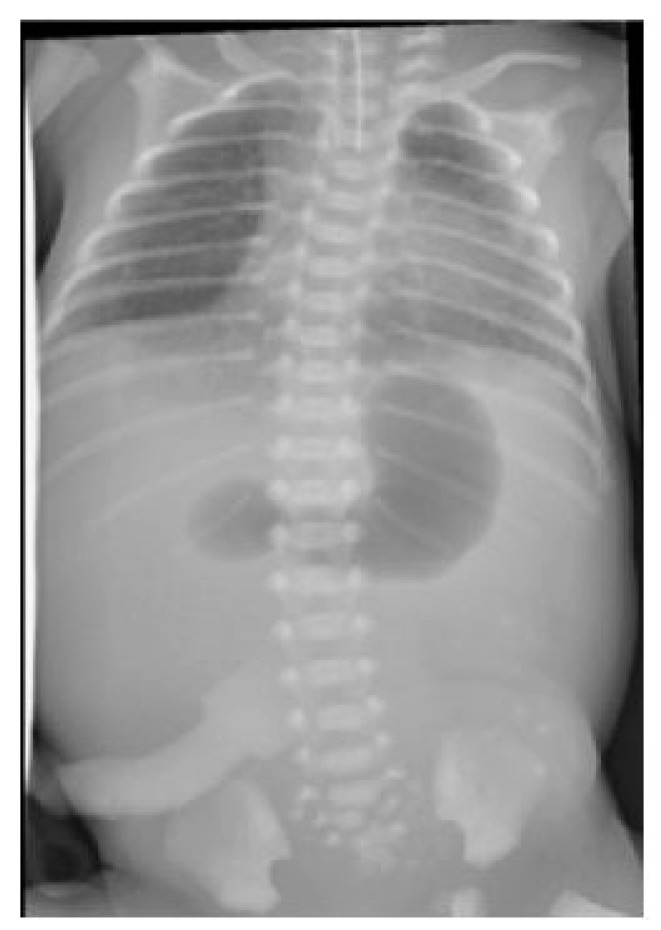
Radiograph demonstrating characteristic double-bubble appearance.
